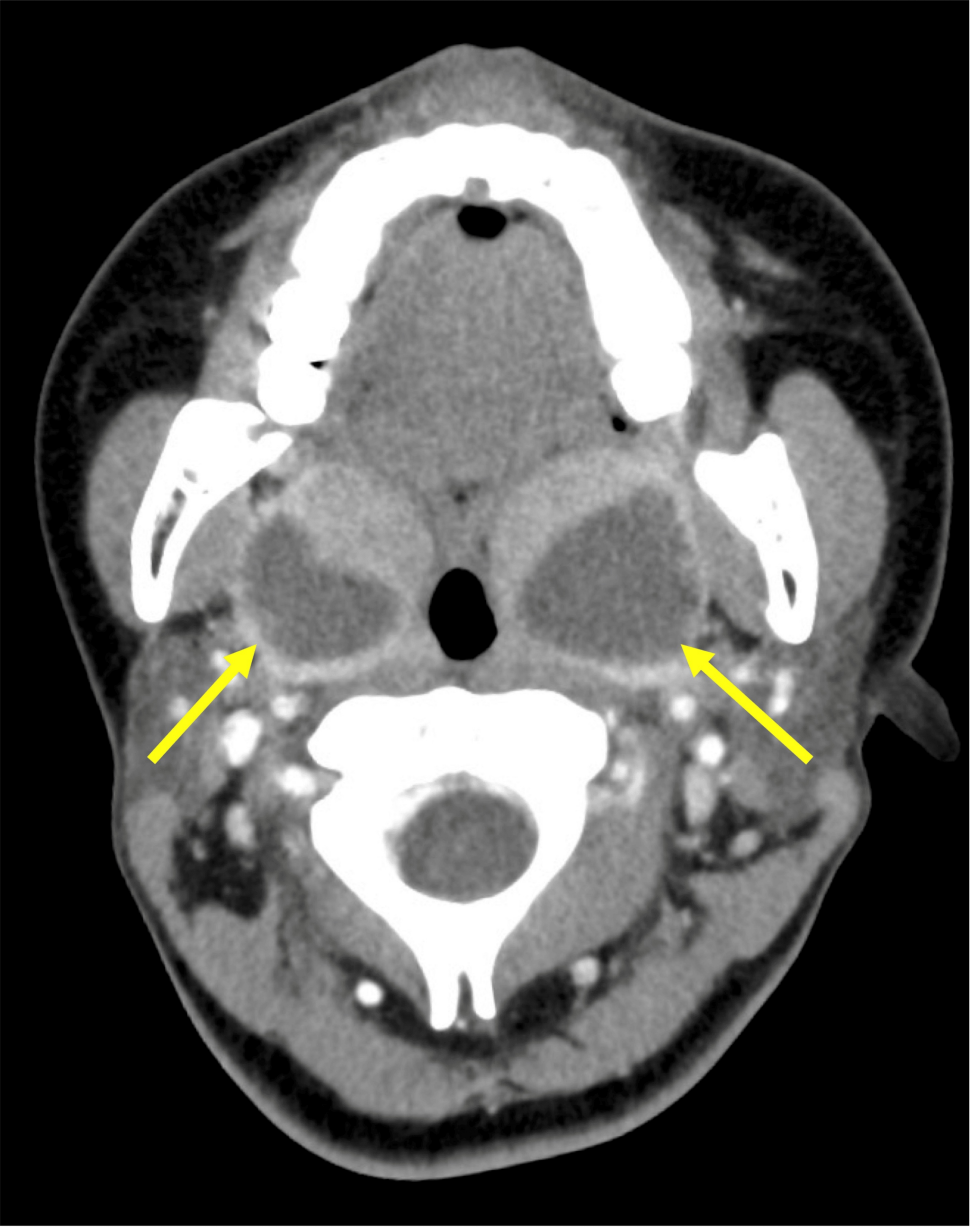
CT findings of bilateral peritonsillar abscess CT showing bilateral peritonsillar abscess (arrow) CT: computed tomography
